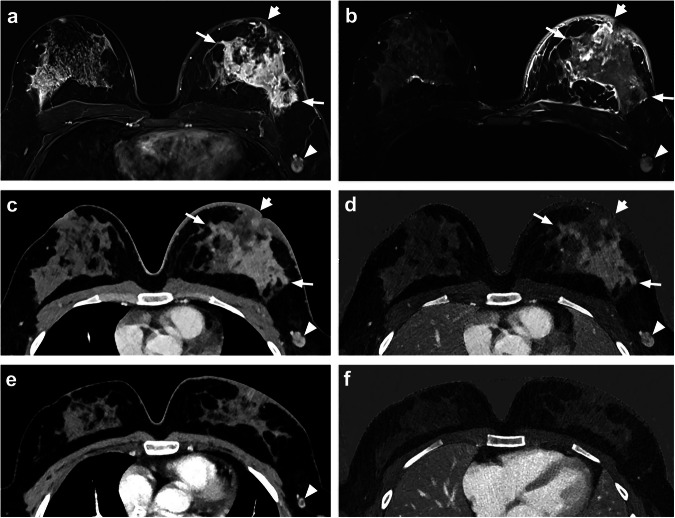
Patient with left-sided multicentric inflammatory breast tumor (long arrow) of no special type, G3, ER-/PR-/HER2+, with cutis infiltration (on the presented slices cutis thickening is indicated with a short wide arrow) and axillary lymph node metastasis (arrowhead) visualized in breast MRI at initial diagnosis with T1w-subtraction after contrast administration (a) and fat-saturated T2w sequence (b). Photon-counting detector computed tomography with monoenergetic reconstruction (c, e) and iodine map (d, f) shows the tumor before NAST (c, d) and with a marked decrease in tumor size and tumor enhancement to a point where it is no longer clearly delineated and a clear reduction in lymph node size following NAST (e, f; f with slightly different angulation, as only e could be angulated for visual presentation to show residual tumor and lymph node in one slice similar to pretherapeutic PCCT). ER, Estrogen receptor; HER2, Human epidermal growth factor receptor; NAST, Neoadjuvant systemic therapy; PCCT, Photon-counting detector computed tomography; PR, Progesterone receptor
